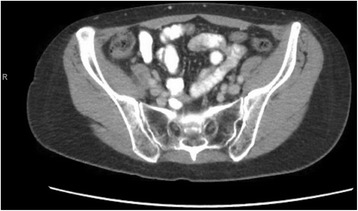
Post neoadjuvant therapy CT scan demonstrating resolution of perirectal lymph node enlargement
